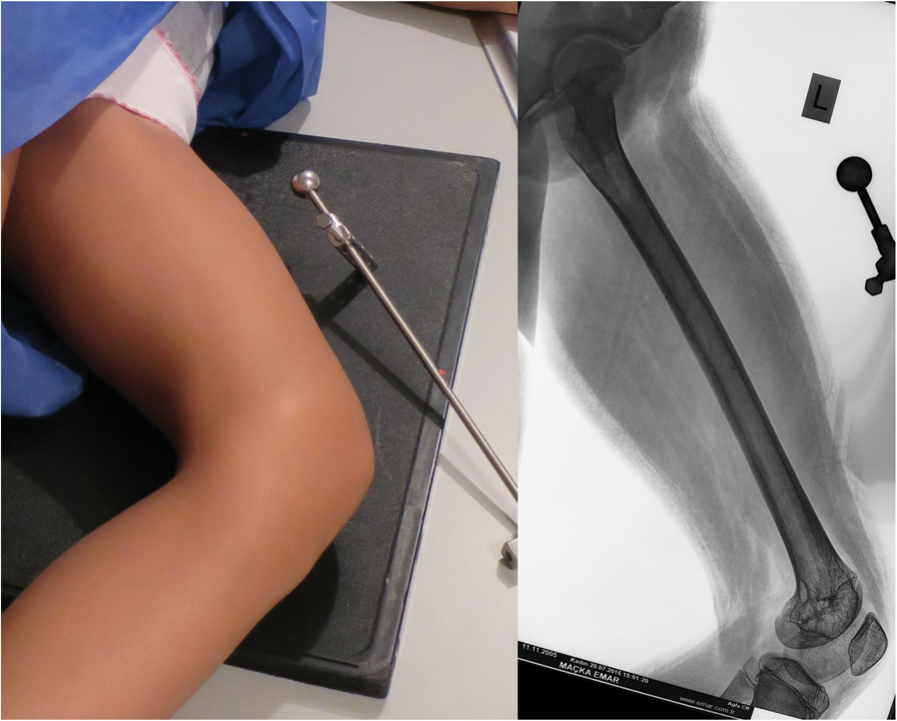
LAT X-ray of the femur. A 20-mm-diameter metallic sphere was positioned at the same distance from both the femur and the cassette for the calculation of the exact size of the femur
